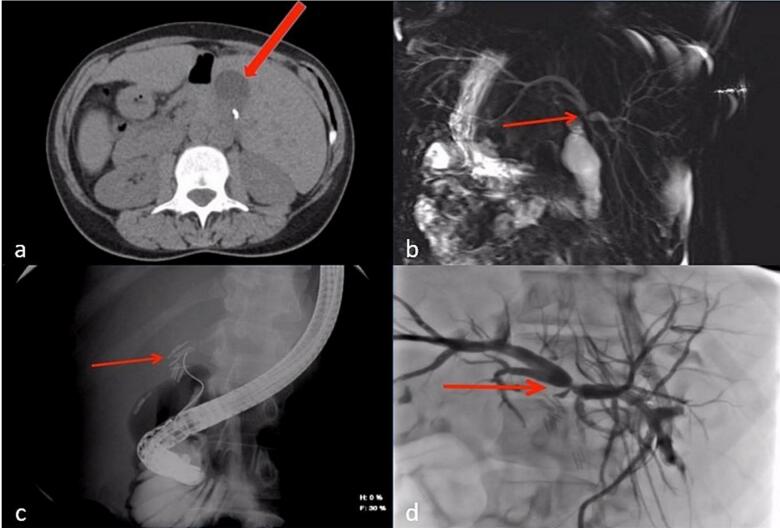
Series of images after the suspected biliary injury. (a) Perihepatic fluid collection on non-contrast CT scan, (b) MRCP showing complete occlusion of the CHD just below the bifurcation (Bismuth-Strasberg Type E3 bile duct injury), (c) ERCP showing complete occlusion of the CHD just below the bifurcation with multiple clips, (d) PTC showing dilation of the biliary tree and occlusion of the CHD (Bismuth-Strasberg Type E3 bile duct injury).•Magnetic resonance cholangiopancreatography (MRCP) was obtained, revealing complete occlusion of the common hepatic duct (CHD) just below the bifurcation of the right and left hepatic ducts (Bismuth-Strasberg Type E3 bile duct injury) [7] (Fig. 2b).•Endoscopic retrograde cholangiopancreatography (ERCP) was attempted, though cannulating the duct was challenging due to her abnormal anatomy. It showed complete occlusion of the CBD and CHD with multiple clips (Fig. 2c).•Percutaneous transhepatic cholangiography (PTC) was also performed, revealing biliary tree dilatation with complete occlusion of the CHD just below the bifurcation (Bismuth-Strasberg Type E3 bile duct injury) [7] with multiple large clips at the occlusion site (Fig. 2d).•A contrast-enhanced CT scan confirmed the appropriate positioning of the PTC catheters and identified abnormal arterial anatomy, including a replaced right hepatic artery (RHA) originating from the superior mesenteric artery (SMA) (Fig. 3a), a replaced left hepatic artery (LHA) originating from the gastroduodenal artery (Fig. 3b), and an accessory left hepatic artery originating from the left gastric artery (Fig. 3c).
